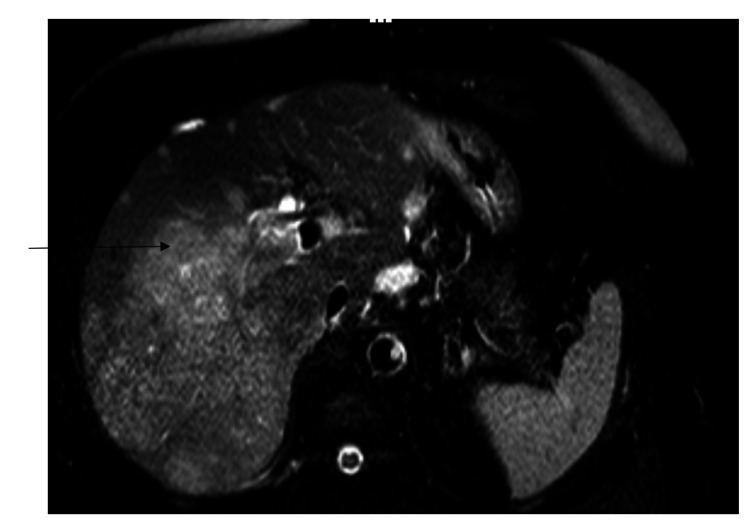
MRI abdomen with and without contrast with an arrow pointing to a lobulated heterogeneously enhanced infiltrative mass involving nearly the entire right lobe of the liver
